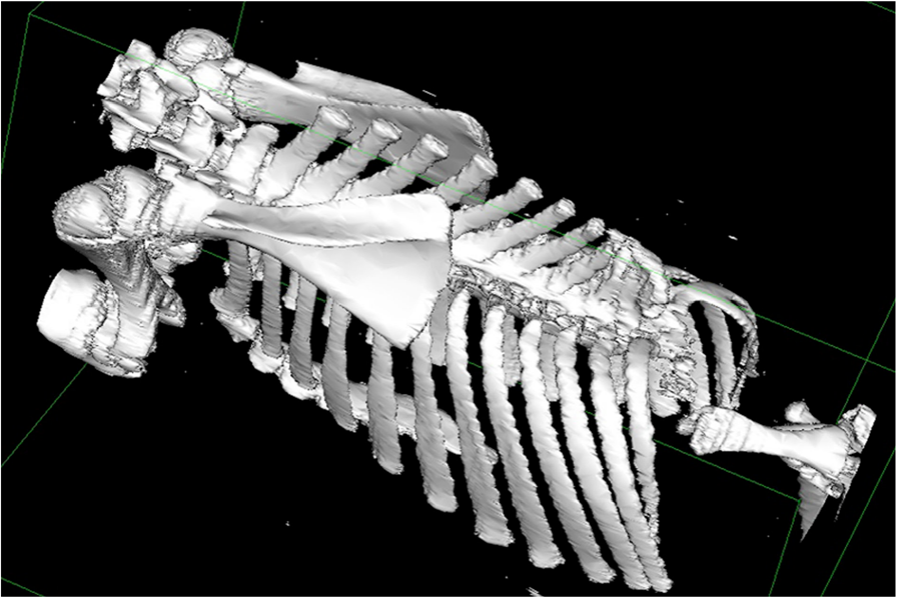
Surface rendered computed tomography image of perosomus elumbis case DK2. Surface rendering^a^ allowing visualisation of the skeletal abnormalities. The caudal thoracic vertebrae are malformed and the lumbar vertebrae are seen as a disorganised bony structure protruding ventrally into the abdomen. The caudal ribs have an uneven course with fusion of the proximal part in the right side.
